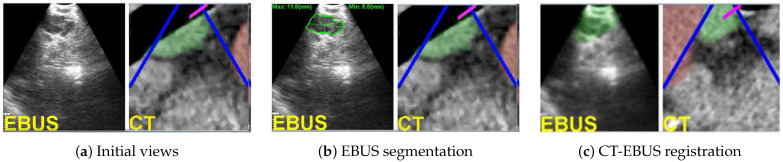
CT-EBUS registration for a station-10 lymph node (case 21405-139). (a) Initial raw EBUS view $I_{\mathrm{US}}$ (left) and corresponding CT-based virtual EBUS view $I_{\mathrm{CT}}^{p_{i}}$ (right). (b) Result after segmenting the nodal ROI $R_{\mathrm{US}}$ in EBUS view $I_{\mathrm{US}}$; the green outline signifies the ROI contour [42]. (c) Registered pair ($I_{\mathrm{US}}$, $I_{\mathrm{CT}}^{p_{o}}$) after final registration; $I_{\mathrm{US}}$ depicts the fused registered CT-based ROI. In all virtual EBUS views, green regions denote nodal ROIs $R_{\mathrm{CT}}$ predefined during planning, whereas red regions represent blood vessels.
